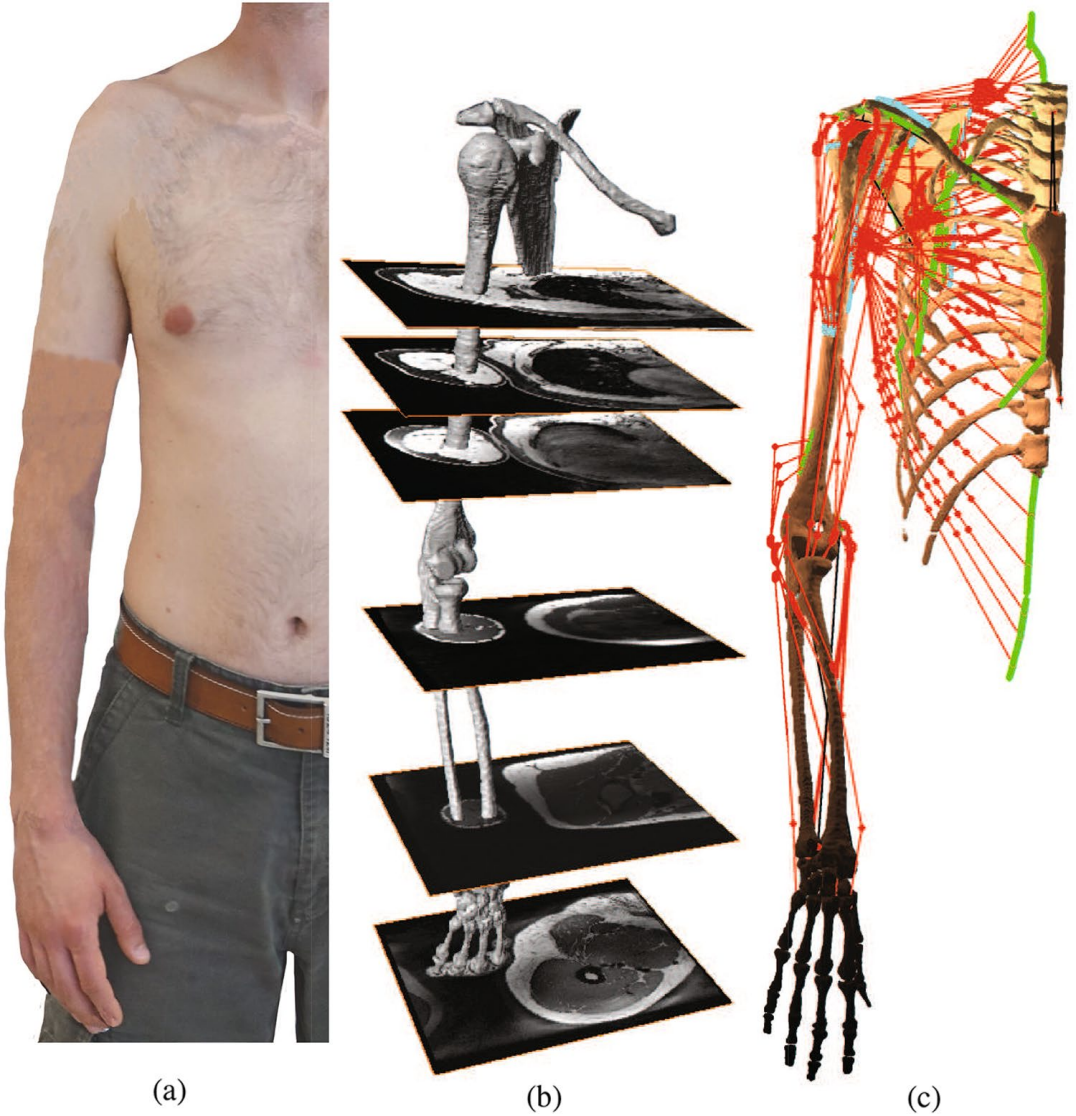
The developed shoulder and elbow model. (a) The anthropometric data of a healthy male subject is used to develop the model. (b) The bone morphologies and muscles origins/insertions are deduced from MRI scans of the same subject. (c) The model consists of thorax, clavicle, scapula, humerus, ulna, radius, and a hand tied to the radius. It has nine DOF represented by eleven generalized coordinates and two holonomic constraints. The model includes 42 muscles that can be represented by up to 20 massless elastic strings. Muscle origins and insertions are denoted by green and blue areas, respectively.
